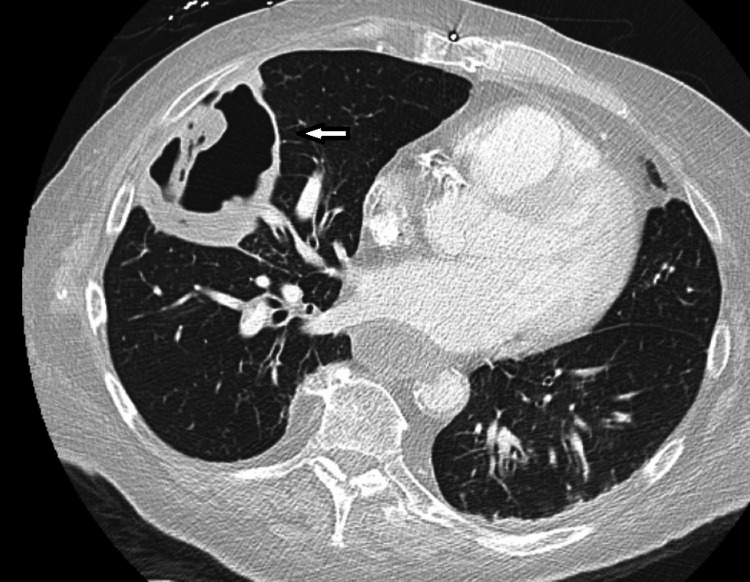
Lung window CT images demonstrating a cavitary lesion with an air–fluid level in the right middle lobe abutting the pleura.
